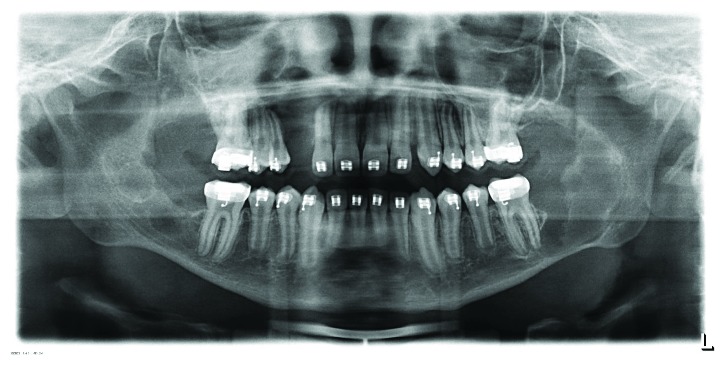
Postoperative control panoramic radiograph (October 2015).
